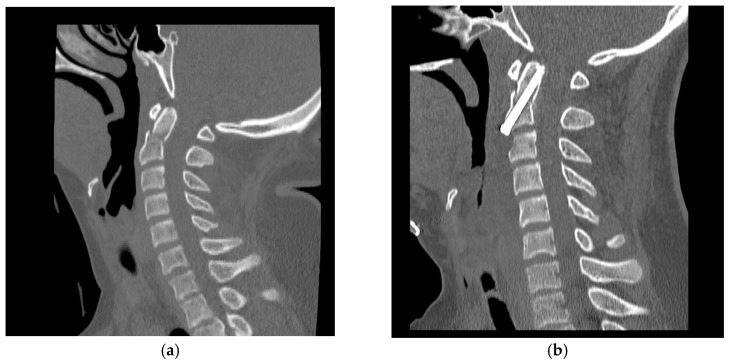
Sagittal CT scans of the cervical spine. (a) Preoperative image showing a type II odontoid fracture with displacement and angulation. (b) Postoperative image demonstrating correct placement of the anterior odontoid screw with anatomical reduction and restoration of alignment. Example from a 17-year-old male patient.
